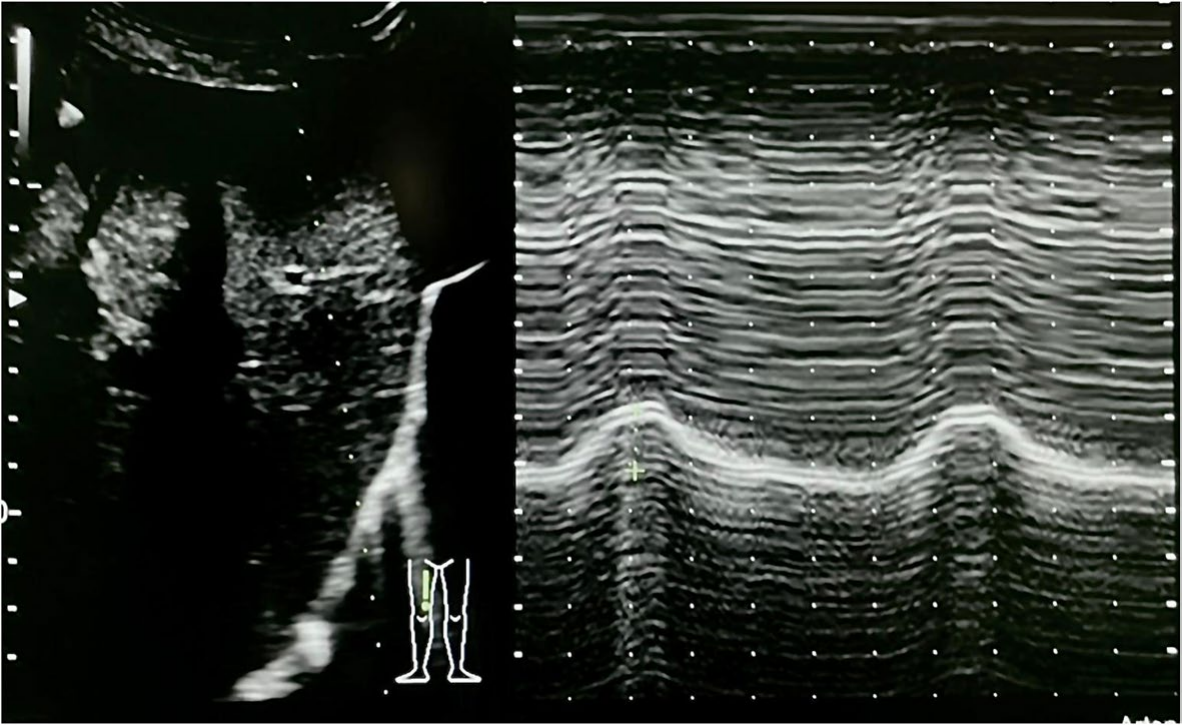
A low-frequency (3.5–5 MHz) ultrasound transducer is used to identify the right hemidiaphragm. The M-mode then is employed to show movements and measure diaphragmatic excursion (cm)
